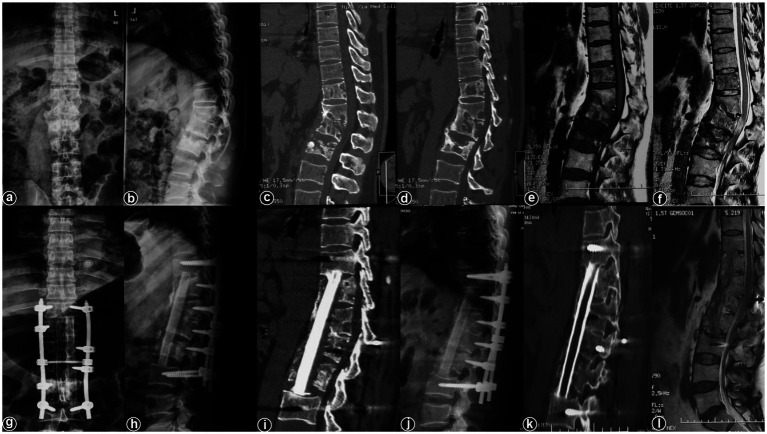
Patient, female, 45 years old. Diagnosis: spinal tuberculosis (T11–L2). Surgical approach: non-intervertebral surgery. (A,B) Preoperative anteroposterior and lateral X-rays of the thoracic spine show narrowing of the T11–L2 intervertebral space and kyphotic deformity. (C,D) Preoperative thoracic CT shows narrowing of the intervertebral spaces and destruction of multiple vertebral bodies. (E,F) Preoperative thoracic MRI reveals multiple abnormal vertebral signals, narrowing of the intervertebral spaces, and spinal cord compression. (G–I) Immediate postoperative anteroposterior and lateral X-rays, and CT of the thoracic spine show short-screw fixation at T11–L2, proper removal of the intervertebral lesion, and satisfactory bone graft placement. (J–L) Three-year postoperative lateral X-rays, CT, and MRI of the thoracic spine show stable internal fixation without loosening or breakage, good bone graft healing, a clear spinal canal, and no recurrence of the local lesion.
